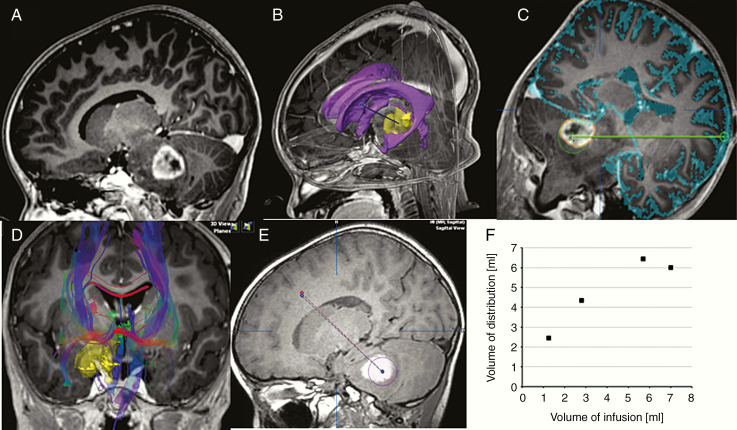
CED infusion in a representative patient. (A) Pre-infusion contrast-enhanced T1-weighted sagittal image. (B) Image showing the complex anatomy. Automatic object creation and fiber tracking can help define the clinical target volume, locate and avoid critical structures during surgical planning, and find the optimal trajectory. Yellow, tumor; purple, ventricle; blue-dotted line, planned catheter tract. (C) Automatic sulcus delineation overlaid on the anatomical images and geometrical visualization of the recommended distance from the catheter tip to avoid structures at risk and minimize the risk of leakage into CSF space. (D) Local detection of fiber bundles in the region of interest provides information about potential leakage pathways, thereby helping to identify an optimal trajectory. (E) Retrospective analysis of catheter placement accuracy. Planned trajectory (red) versus the actual catheter position (blue). Distribution of tracer agent (yellow outline). (F) The volume of distribution at each MRI time point plotted against the volume of infusion. *V*_d_/*V*_i_ was almost 2 at the beginning of infusion, but gradually slowed with *V*_d_ plateauing at 6–7 mL.
